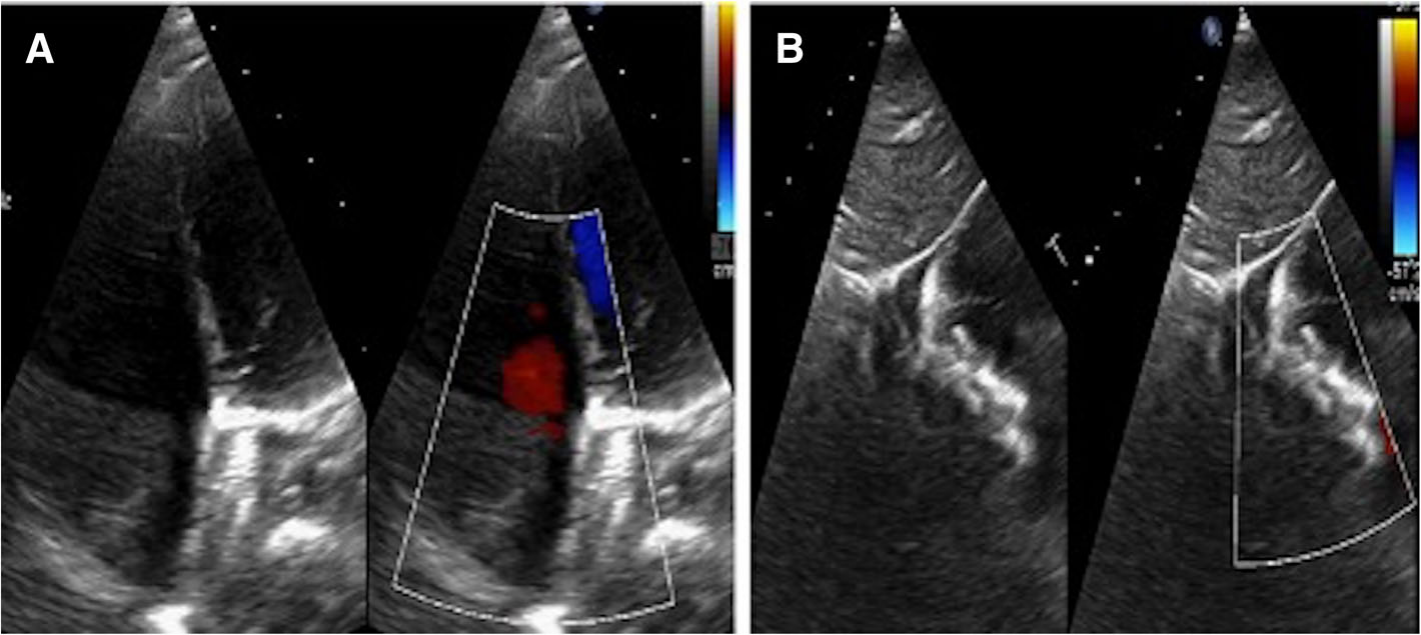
Final transthoracic echocardiographic images confirming the proper positioning of the device across the interatrial septum in apical 4-chamber (A) and subcostal (B) views
